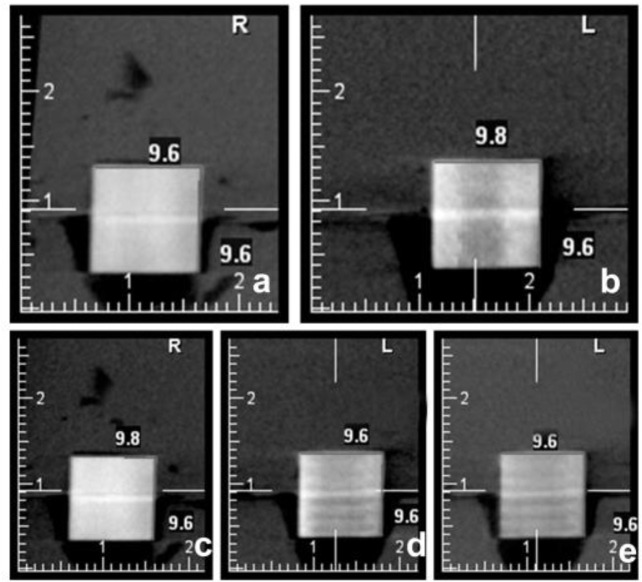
Cross-sectional images of CBCT scans: (a) left position; (b) center; (c) right position; (d) anterior position; (e) posterior position
